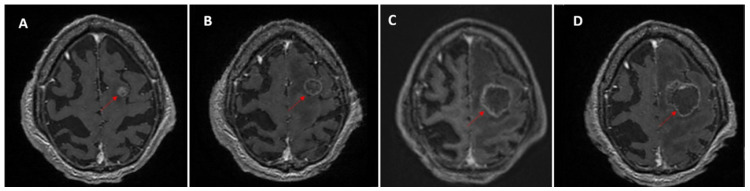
Longitudinal MRI of a left frontal brain metastasis (red arrows) in a patient with lung cancer treated with stereotactic radiotherapy (SRT, 35 Gy/5 fractions). (A) Baseline scan before SRT shows an enhancing lesion. (B–D) Post-treatment follow-up demonstrates dimensional increase with irregular, thin margins and persistent enhancement over time.
